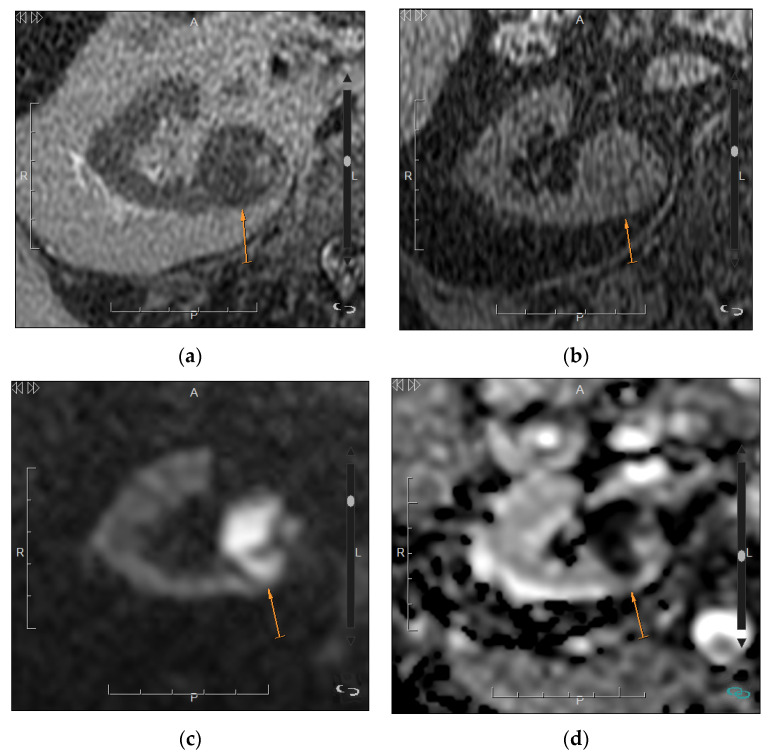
Papillary renal cancer in a 66-year-old man. (a) Axial T2-weighted MRI shows an almost complete low-signal mass (arrow) in a small kidney with a thin cortex due to chronic renal disease; (b) Axial fast spin-echo T1-weighted MRI shows isointensity of the mass without macroscopic fat (arrow); (c) Axial diffusion-weighted image with high b = 1000 (d) and ADC map show areas of restricted diffusion (arrow). No sequences after the administration of the contrast agent were performed due to the chronic kidney disease. Scale size: 5 cm.
